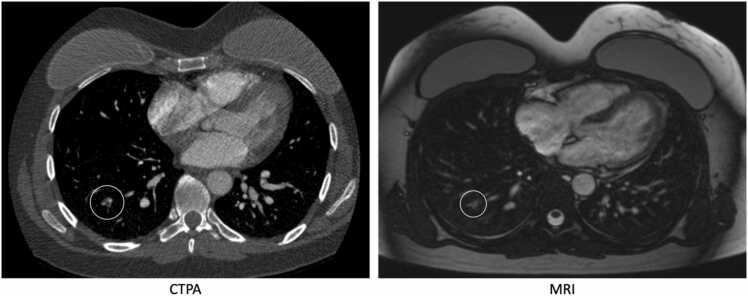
45 years old female, 16 hours and 20 minutes between CTPA and MRI. Missed subsegmental emboli in RLL on MRI by R1 and R2.
